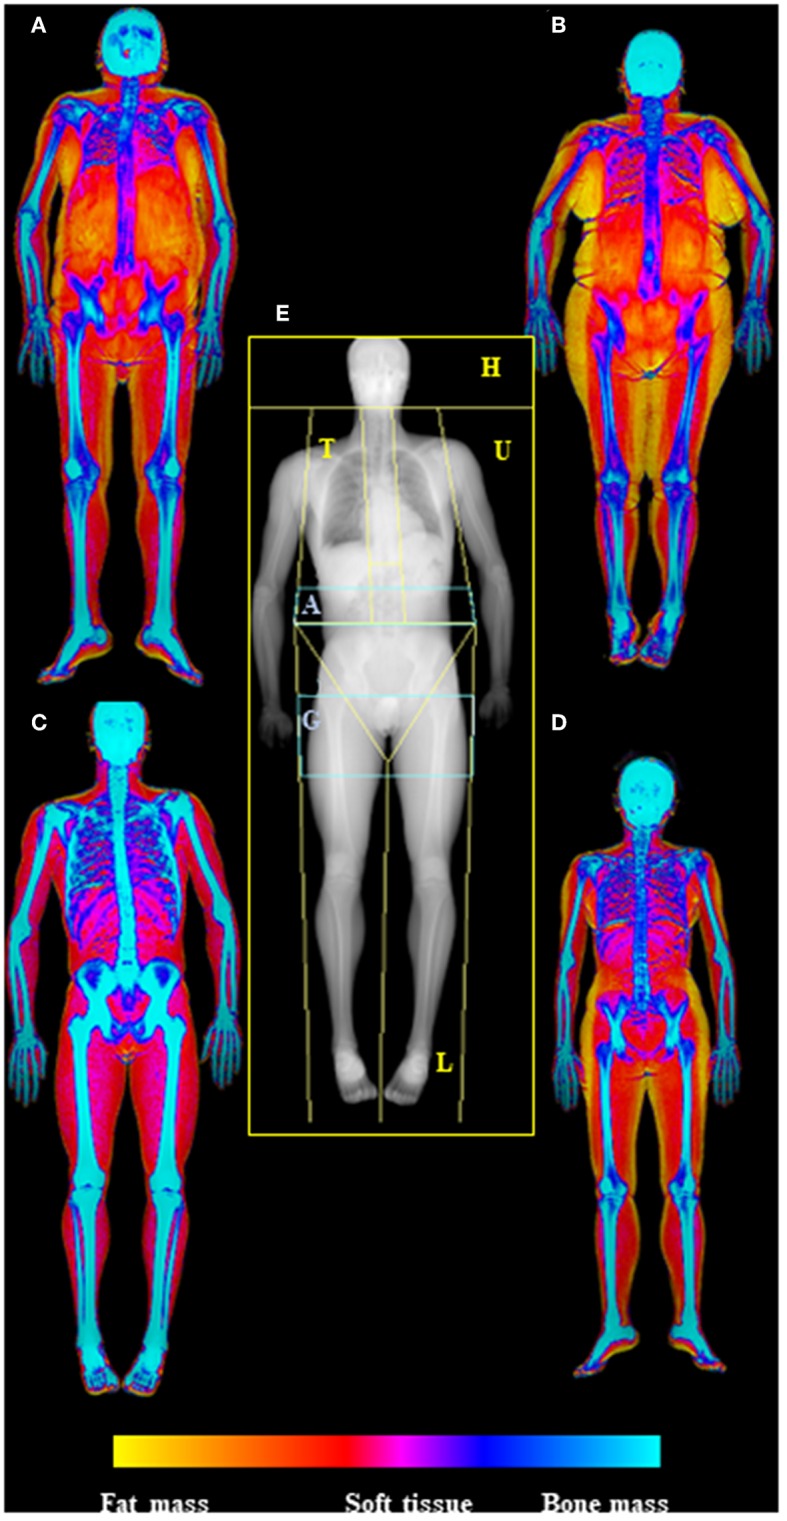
Dual-energy X-Ray absorptiometry (DXA) examination of body composition. In the center of the picture (E) is represented the skeletal map of whole body scan by DXA highlighting the standard ROIs specifics for body composition assessment (head—H, trunk—T, upper limbs—U, lower limbs—L), with the two regions at “high metabolic significance” representing by gynoid (G) and android (A) regions. On the side are depicted the soft tissue maps of whole body DXA scan (from fat mass—yellow—to bone mass—blue); in particular on the left are visualized old (A) and young (C) males (respectively upper and lower), while in the right old (B) and young (D) females (respectively upper and lower), highlighting the increase of fat mass in aging. Images are kindly provided by IRCCS Rizzoli Orthopedic Institute, Unit of Diagnostic and Interventional Radiology (2019).
